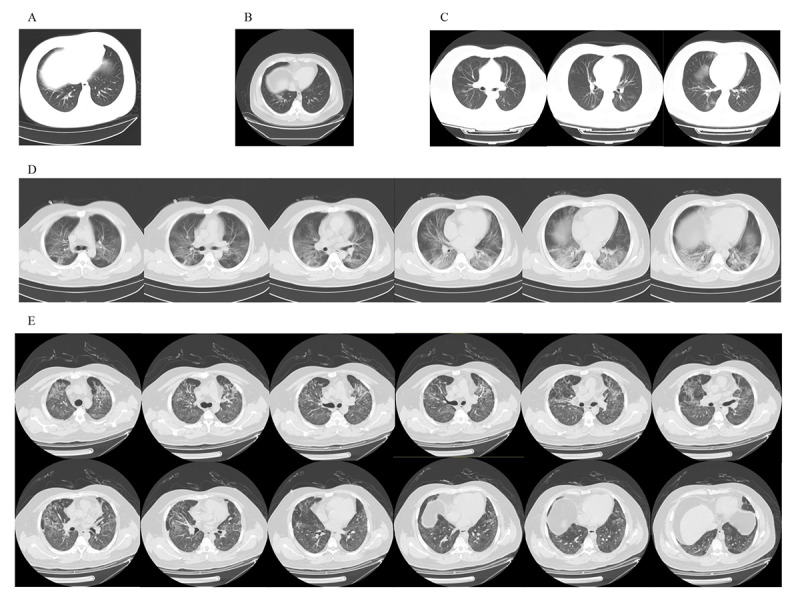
Chest computed tomography (CT) images of Patient No. 4 showing small patchy shadows on the lower right lung on day 1 (A) and day 3 (B) after symptom onset. Chest CT images showing bilateral ground-glass opacities on day 6 after symptom onset (C) and bilateral large infiltrative shadows with partial consolidation on day 10 after symptom onset (D). Chest CT images showing bilateral diffuse reticular changes and fibrous stripes on day 45 after symptom onset (E).
